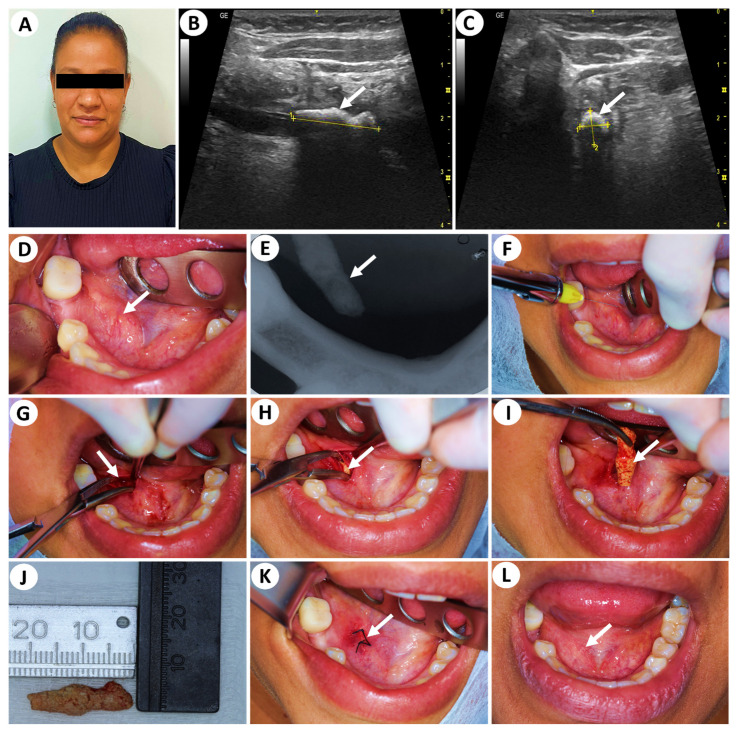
Patient profile (A), ultrasound exams (B,C), initial intraoral aspect (D), initial radiographic exam (E), sequential approach for removal of giant sialolith (F–K) and final aspect after surgical treatment (L). In A, absence of abnormalities extraoral. In (B,C) (white arrows), oval and hyperechoic image located in the distal topography of the right of Wharton’s duct. In (D), ovoid and well-defined submucosal swelling with normal color and texture (white arrow). In (E), radiographic aspect showing a mass along the internal surface of the right mandible (white arrow). In (F), local anesthesia (using articaine hydrochloride with epinephrine; DFL Indústria e Comércio, Rio de Janeiro, RJ, Brazil) technique. In (G), incision and divulsion (white arrow). In (H), divulsion and dissection of tissues to locate the giant sialolith (white arrow). In (I), removal of the giant sialolith (white arrow shows the giant sialolith). In (J), the length (21 mm) and the width (9 mm) of the giant sialolith. In (K), sutured tissues using 4-0 silk (white arrow; Ethicon, Johnson and Johnson do Brasil, São José dos Campos, SP, Brazil). In (L), absence of swelling and normal appearance of the oral mucosa seven days postoperatively (white arrow).
